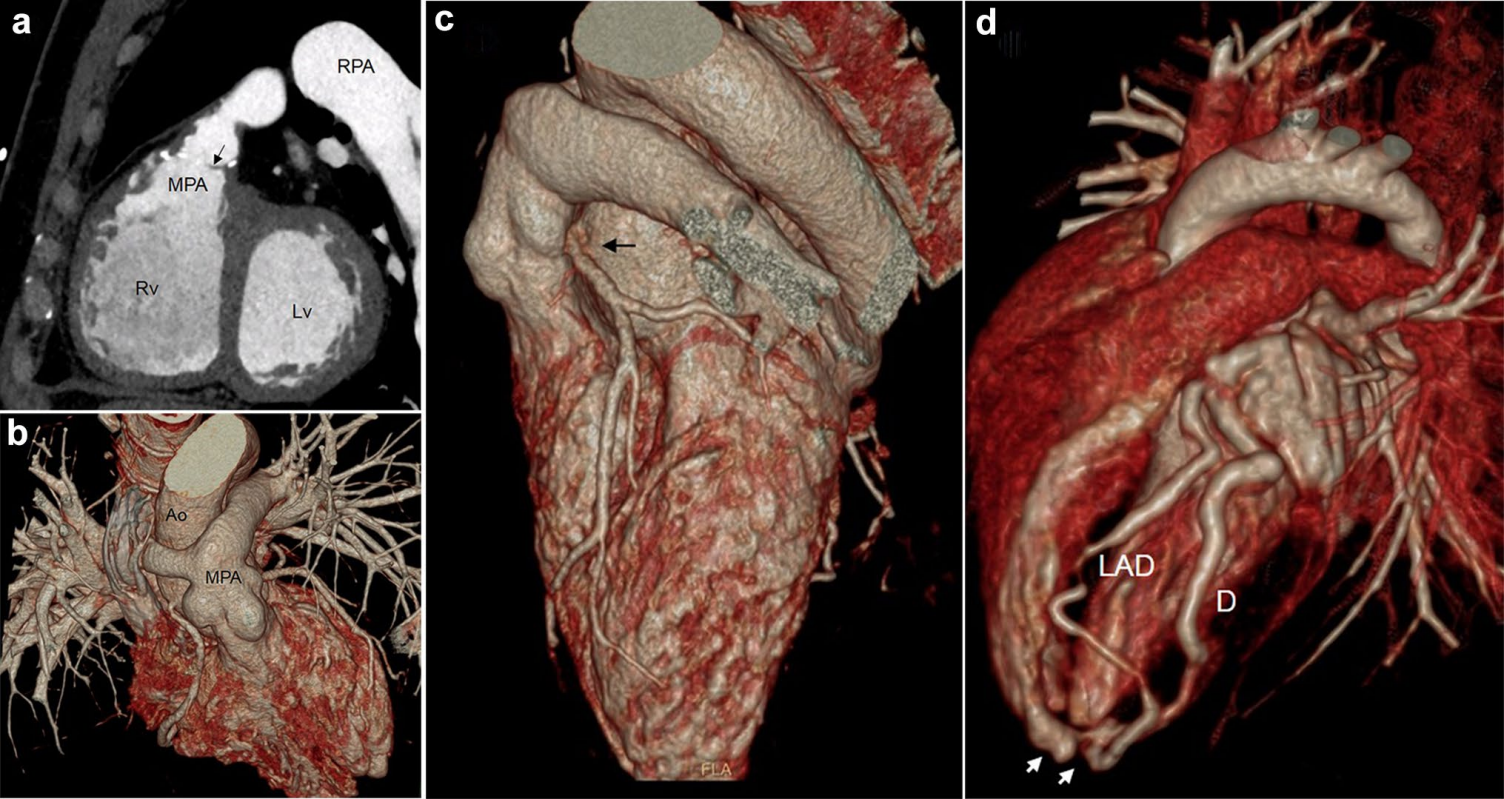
31-year-old male with a repaired ToF. CCT MPR image, RVOT sagittal plane: infundibular and pulmonary stenosis (a), note the anatomical detail of valvular cusp (arrow). 16-year-old female with a cTGA after Arterial Switch operation. CCT 3D volume rendering images demonstrate high resolution post-surgical anatomy (b), LCA reimplantation kinking and stretching (black arrow) is well depicted (c). 2-year-old child with coronary artery fistula. CCT 3D volume rendering image optimally shows the fistula (white arrows) between LAD artery and Rv chamber (d). *Ao* Ascending aorta, *CCT* Cardiovascular computed tomography, *cTGA* Complete transposition of the great arteries, *3D* Tridimensional, *D* Diagonal artery, *LAD* Left Anterior descending artery, *LCA* Left coronary artery, *Lv* Left ventricle, *MPA* Main pulmonary artery, *MPR* Multiplanar Reformation, *RPA* Right pulmonary artery, *Rv* Right ventricle, *ToF* Tetralogy of Fallot
